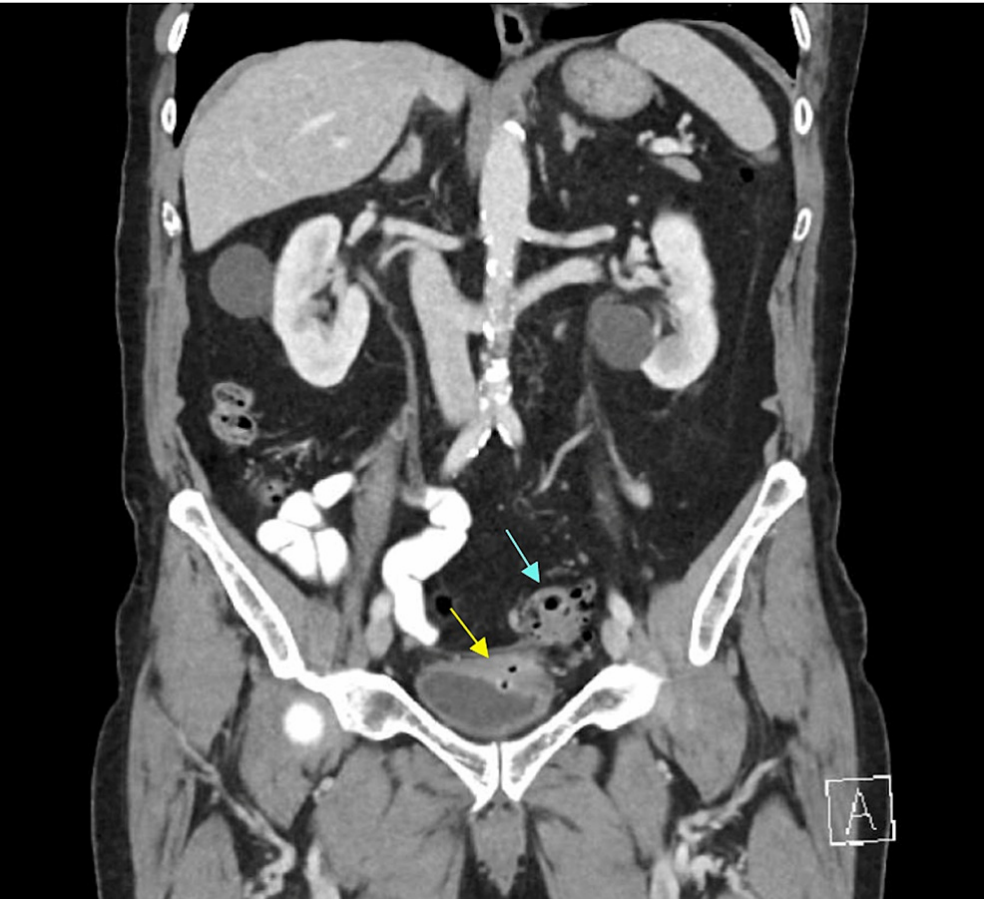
Coronal computed tomography of the abdomen and pelvis with intravenous and oral contrast (Gastrografin). The image shows gas locules within the bladder (yellow arrow) and adjacent sigmoid diverticulitis (blue arrow) consistent with colovesical fistula.
